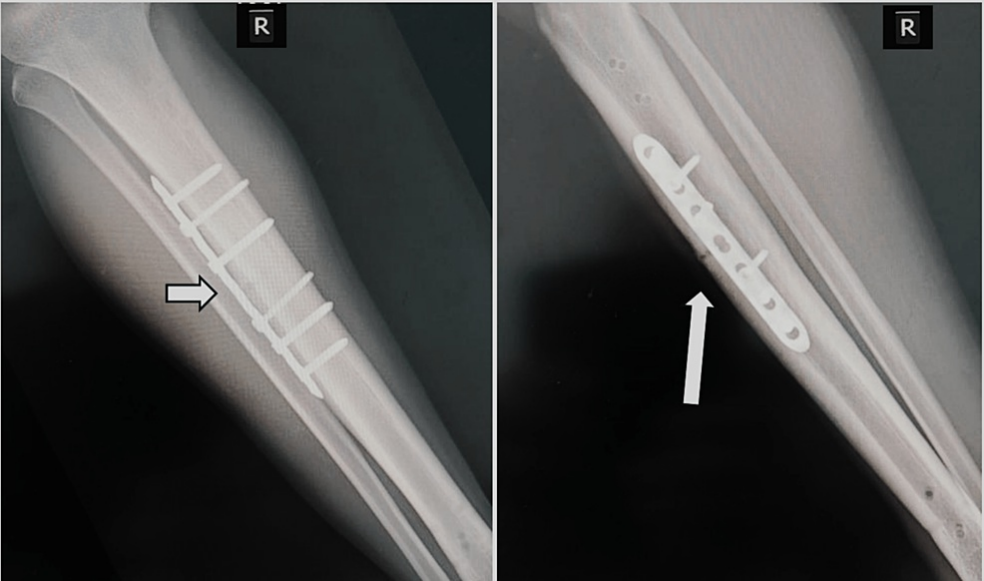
X-ray of the revised nonunited right tibia The images show the tension plate and autologous iliac crest graft on the right tibia with adequate bone healing six months postoperatively
